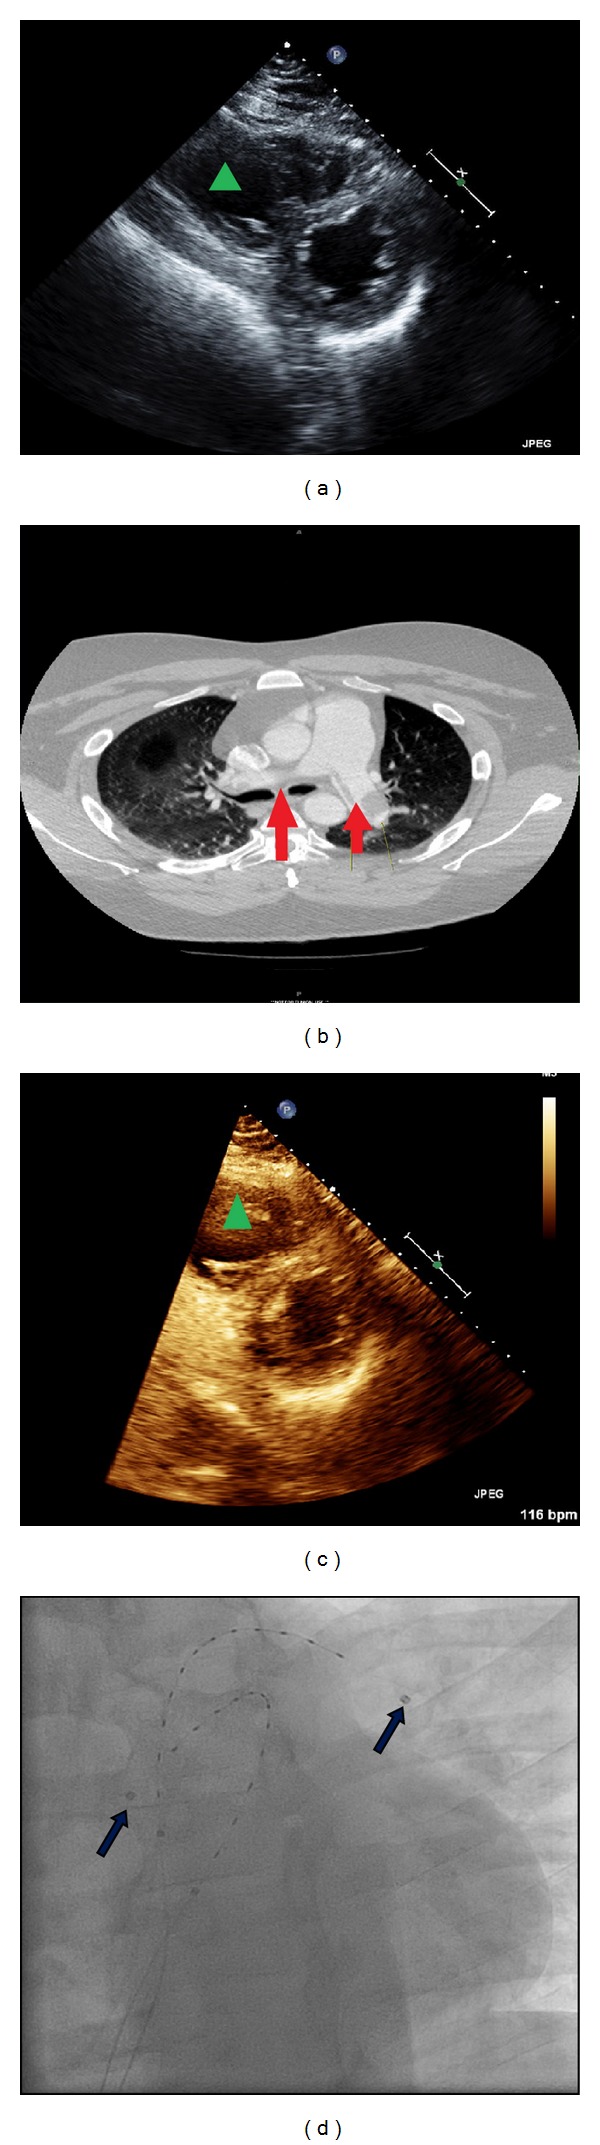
(a) The green triangle is located in the markedly dilated right ventricle. (b) The red arrows point at saddle emboli. (c) The echocardiogram shows improvement in the size of right ventricle. (d) The fluoroscopy confirms the placement of the UAT catheter in both main pulmonary arteries (blue arrows).
